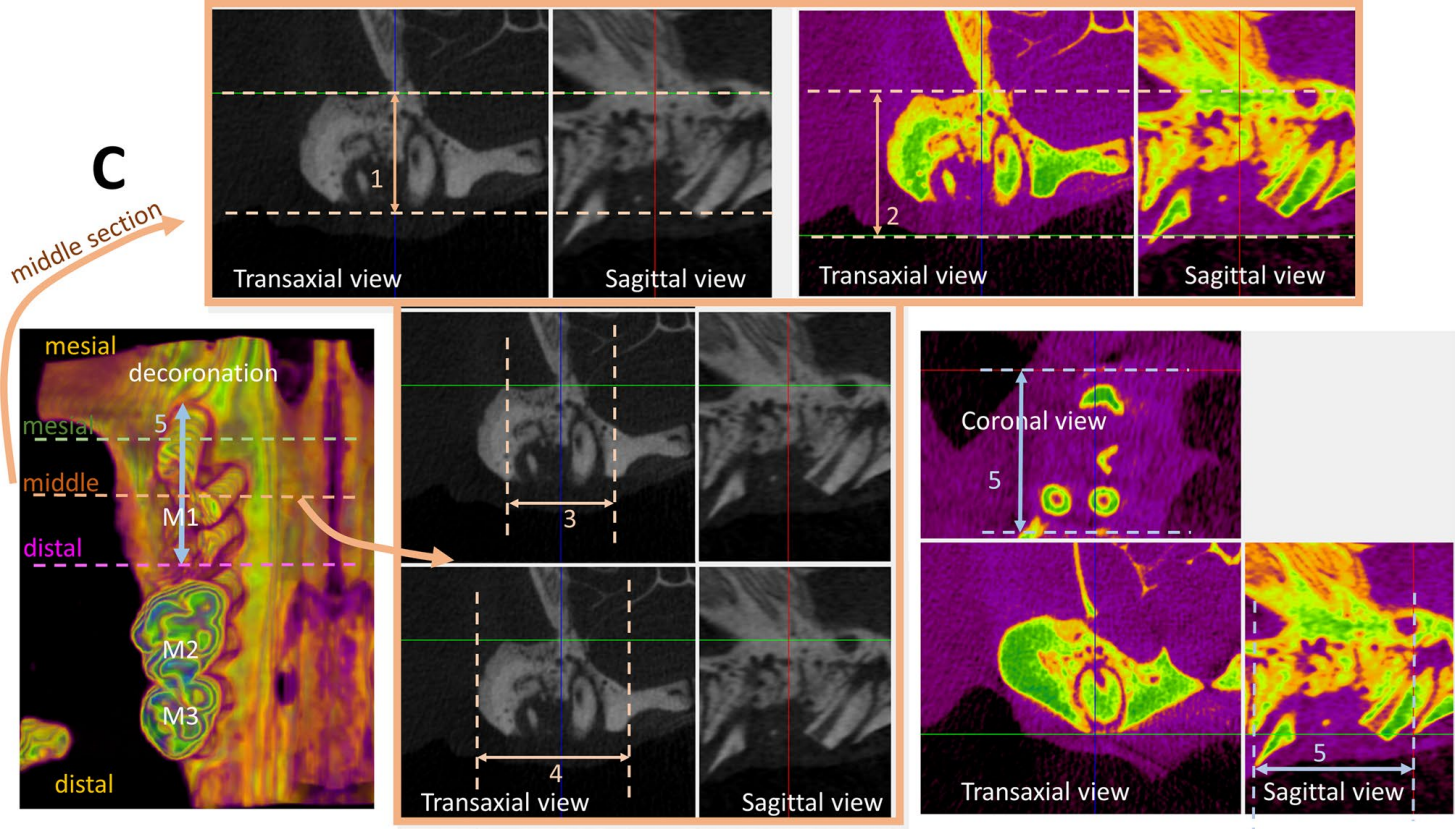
Micro-computed tomography assessments of alveolar bone and soft tissue dimension. (A) To evaluate the effectiveness of hard and soft tissue preservation of alveolar ridge dimension associated with the decoronation procedures, thirteen parameters were measured at maxillary first molar (M1) sites including (1) distal buccal bone height, (2) distal palatal bone height, (3) distal maximum soft tissue height, (4) middle buccal bone height, (5) middle palatal bone height, (6) middle maximum soft tissue height, (7) mesial buccal bone height, (8) mesial palatal bone height, (9) mesial maximum soft tissue height, (10) 3D bone contour volume*, (11) distal maximum alveolar ridge width, (12) middle maximum alveolar ridge width, and (13) mesial maximum alveolar ridge width. (B) To evaluate the influence of decoronation on the periodontal conditions of maxillary second molar (M2) and third molar (M3), the distances from marginal bone to cemento-enamel junction (CEJ) including (1) bM2mCEJ: M2 mesiobuccal CEJ to bone; (2) bM2dCEJ: M2 distobuccal CEJ to bone; (3) bM3mCEJ: M3 mesiobuccal CEJ to bone; (4) bM3dCEJ: M3 distobuccal CEJ to bone; (5) pM2mCEJ: M2 mesiopalatal CEJ to bone; (6) pM2dCEJ: M2 distopalatal CEJ to bone; (7) pM3mCEJ: M3 mesiopalatal CEJ to bone; (8) pM3dCEJ: M3 distopalatal CEJ to bone; and (9) the distance from CEJ to mesial furcation of M2 (M2mf) were measured. (C) To provide reference for alveolar critical size defect dimensions in a standardized and discriminative manner, five parameters were measured at the decoronated M1 site, including (1) middle bone height, (2) middle tissue height, (3) middle alveolar crestal width, (4) middle maximum bone width, and (5) mesio-distal socket length. *Three-dimensional (3D) bone contour volume was calculated on either side by adding up consecutive surfaces of interest on the transaxial section mesio-distally throughout the M1 site.
